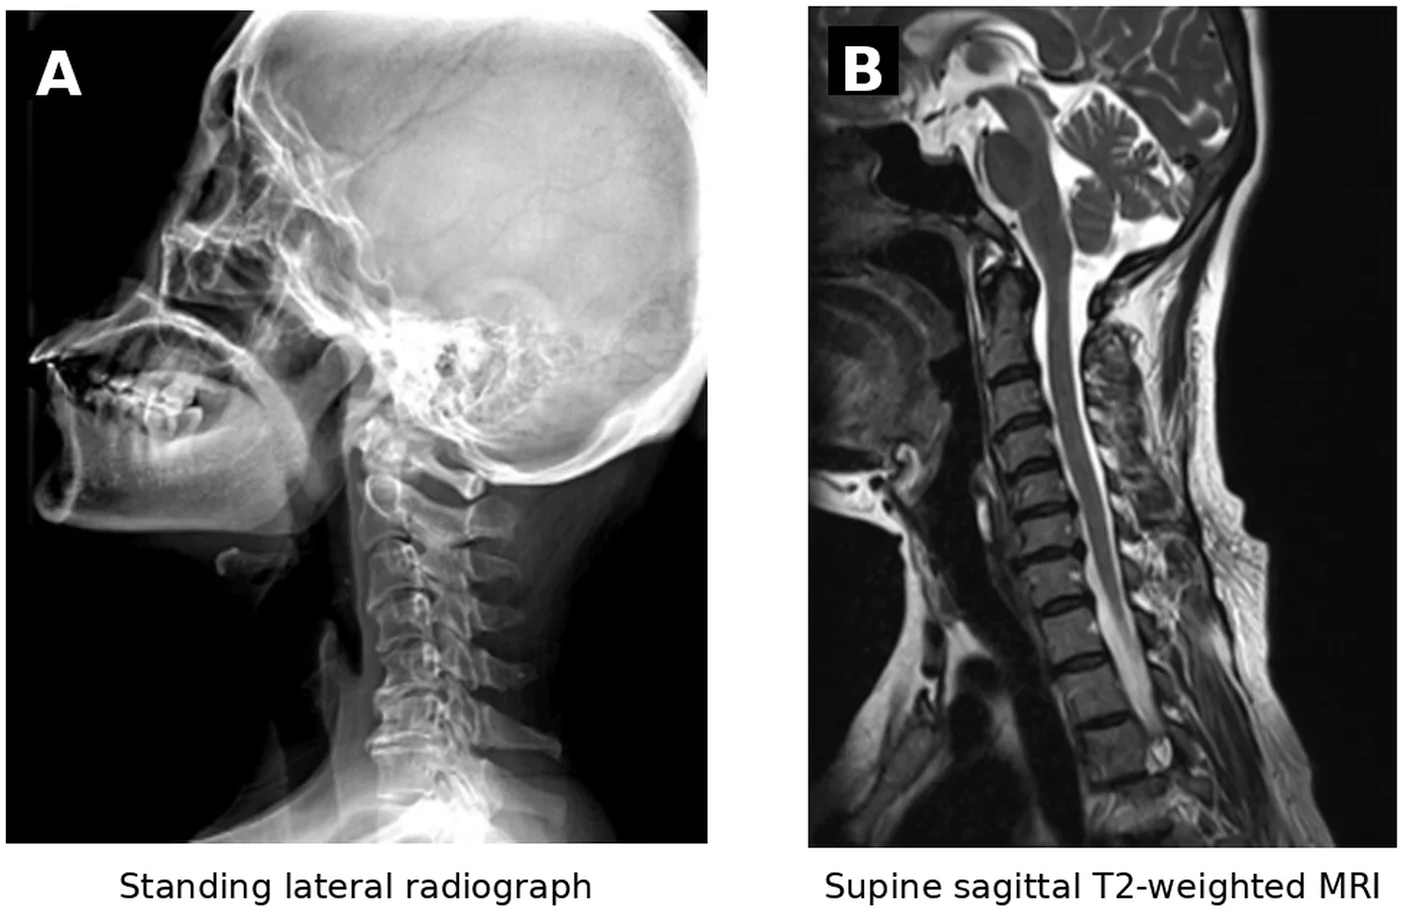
Representative assessment of posture-related angular change (PAC) at C5–6. A: Standing lateral cervical radiograph. B: Supine mid-sagittal T2-weighted MRI. The local C5–6 Cobb angle was measured on each image using tangent lines to the inferior endplates of C5 and C6. Absolute PAC was defined as |standing radiographic Cobb angle—supine MRI Cobb angle|. Patients with |PAC| ≤ 5° were classified as low-PAC/relatively stiff, whereas those with |PAC| > 5° were classified as high-PAC/relatively mobile. PAC is an operational posture-related angular measure and is not equivalent to dynamic flexion-extension mobility.
